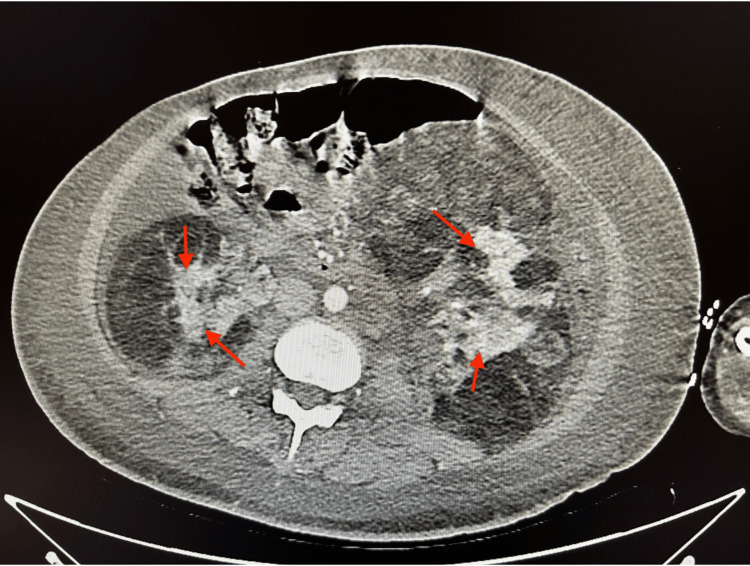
Abdominal CT visualizes multiple renal angiomyolipomas bilaterally CT: Computed tomography; Red arrows: Renal angiomyolipomas
